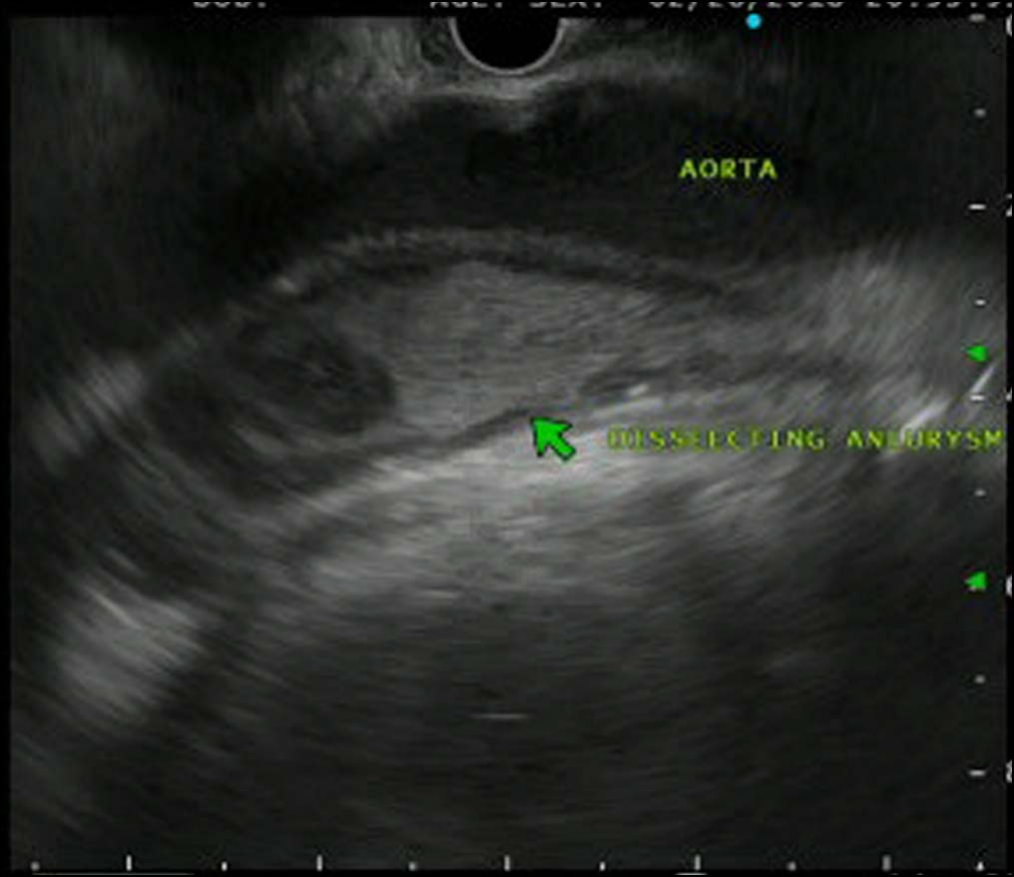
Type B aortic dissection as seen on endoscopic ultrasound.
